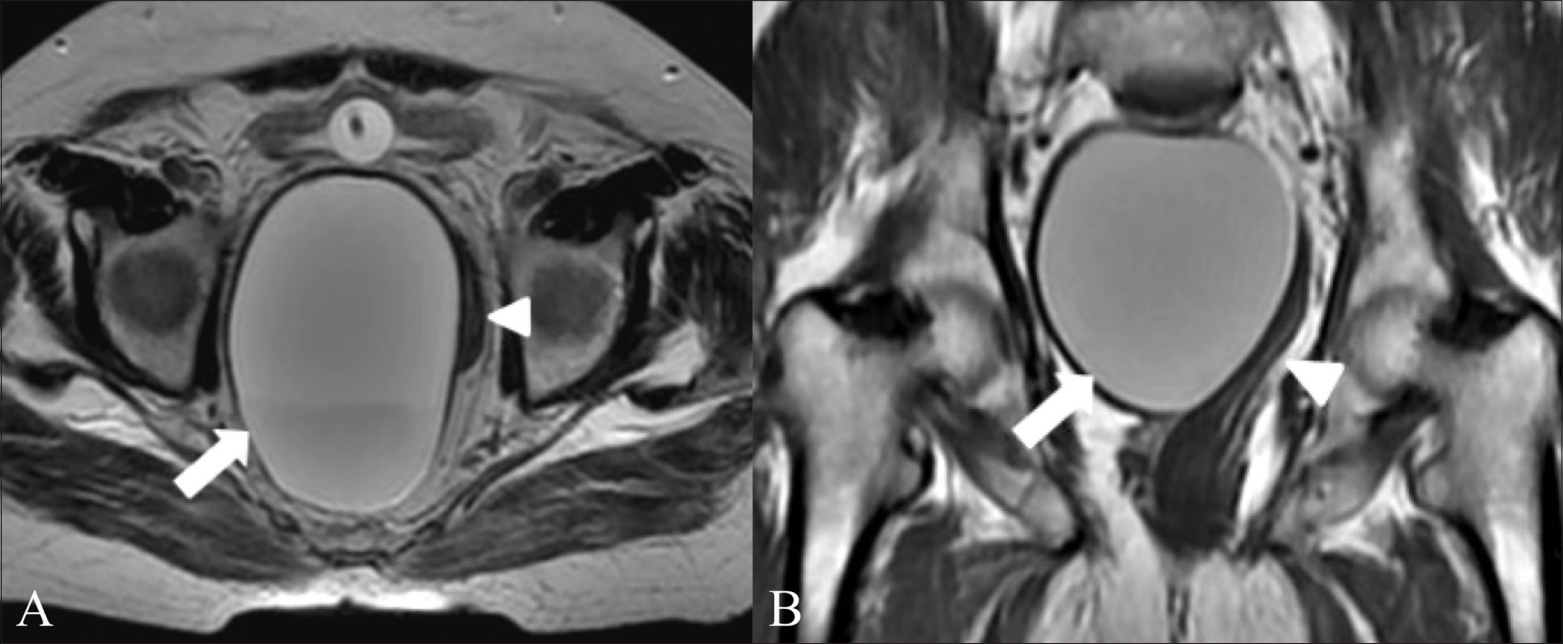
Axial T2W (A) and coronal T1W (B) MRI images show a large, hyperintense tailgut cyst (arrow) along the right margin of the rectum (arrowhead), which is displaced to the left
